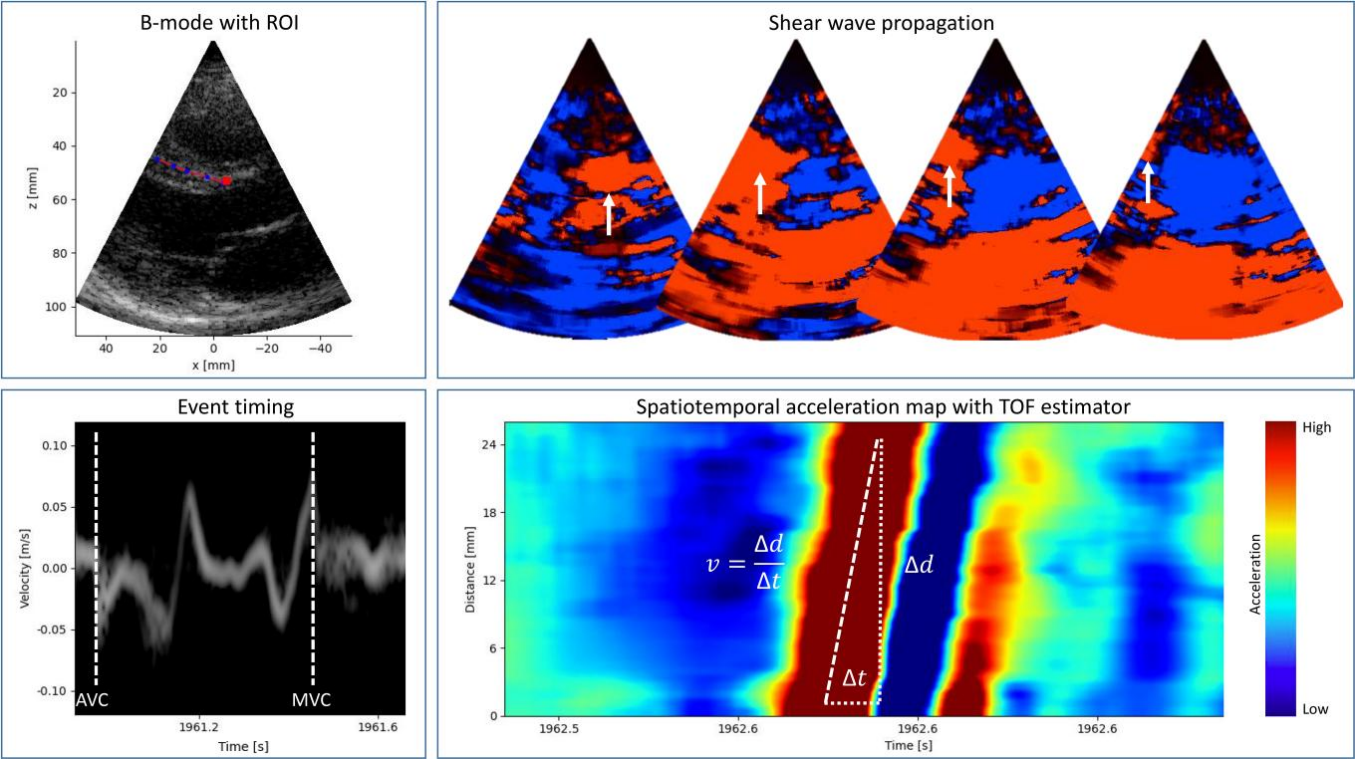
SWE. Top left panel: B-mode image from a PLAX with ROI in the IVS (dashed line) and marker for pulsed tissue Doppler in the basal septum. Top right panel: Analysis of the raw IQ data by estimating tissue motion by tissue Doppler imaging. White arrows follow an AVC wave propagating in the IVS. Bottom left panel: Tissue Doppler curve for identifying shear waves triggered by intrinsic events in the cardiac cycle. Bottom right panel: Spatiotemporal acceleration map measuring shear wave velocity by application of a time-of-flight estimator. AVC, aortic valve closure; IQ, in-phase and quadrature data components; MVC, mitral valve closure; ROI, Region of interest; TOF, time-of-flight.
